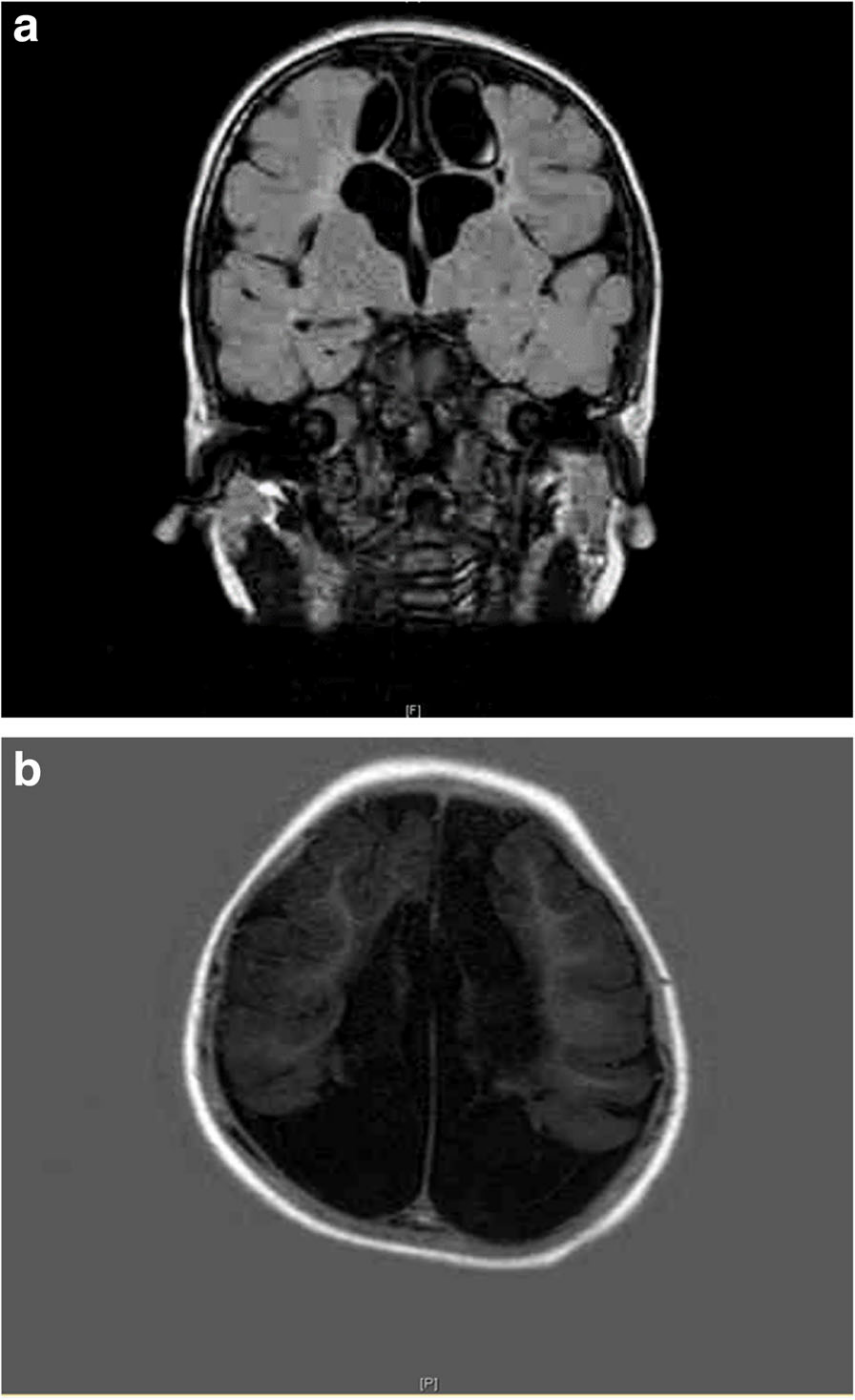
Postnatal magnetic resonance imaging of the brain (a) coronal T2- weighed FLAIR image and (b) axial T1- weighed image of second baby at 1.5 years of age showing microcephaly, extensive loss of supratentorial brain parenchyma replaced by multiple cystic spaces, more severely at bilateral peritrigonal and parafalcial regions, with ex-vacuo dilatation of bilateral ventricles, also severe thinning of the corpus callosum
